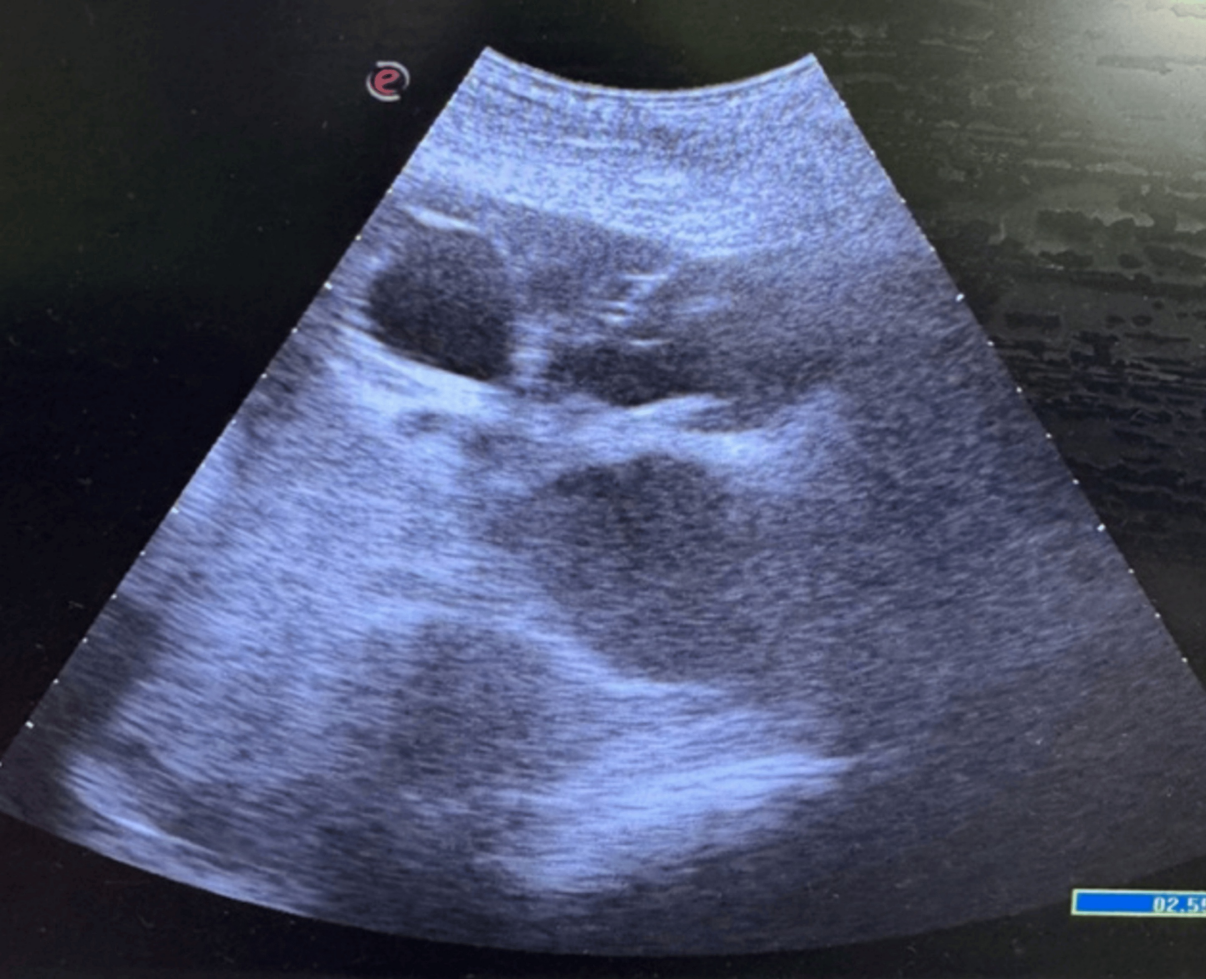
Ultrasound-guided puncture of a dilated pelvicalyceal system
